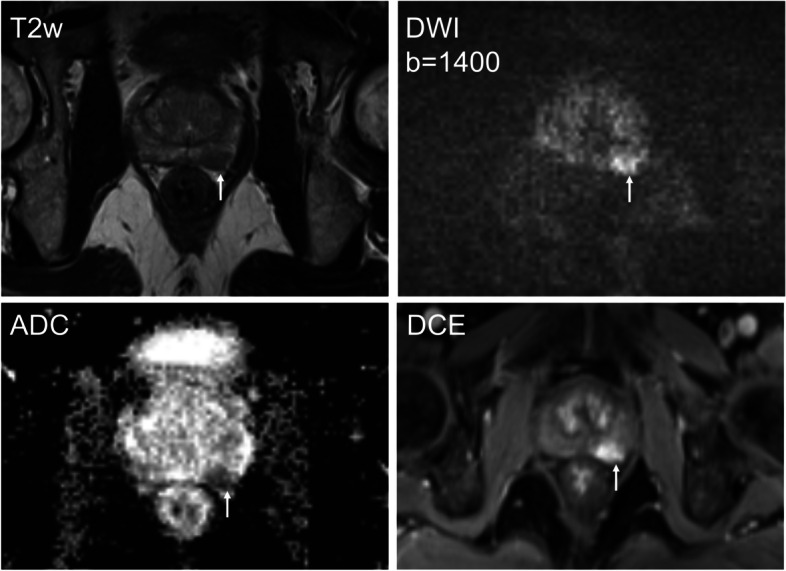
MR images in a 63-year-old man with a prostate-specific antigen (PSA) level of 7.39 ng/ml. The prostate volume measured by MRI was 42.42 ml, and the PSA density was 0.174 ng/ml^2^. Multiparametric MRI demonstrated a lesion measuring 1.1 cm in the left posterior peripheral zone midgland (arrow) and ill-defined margins on T2-weighted images with severe restricted diffusion and early contrast enhancement; Prostate Imaging Reporting and Data System (PI-RADS) category 4. On subsequent cognitive MRI-target biopsy, all 3 targeted cores demonstrated Gleason score (GS) 4 + 5 with maximum tumor core involvement of 57%. Five out of 12 systematic biopsy (SB) cores were positive for prostate cancer with the highest GS 4 + 5 and maximum tumor core involvement of 26%. At a risk threshold of 60%, both the MRI model (predicted risk: 67.6%) and the combined model (predicted risk: 65.6%) would have resulted in obviating SB. The predicted risk for the clinical model was 35.7%. The patient would have undergone unnecessary SB based on the clinical model and PI-RADS 5 strategy. ADC, apparent diffusion coefficient; DWI, diffusion-weighted imaging; DCE, dynamic contrast-enhanced; T2w, T2-weighted imaging
